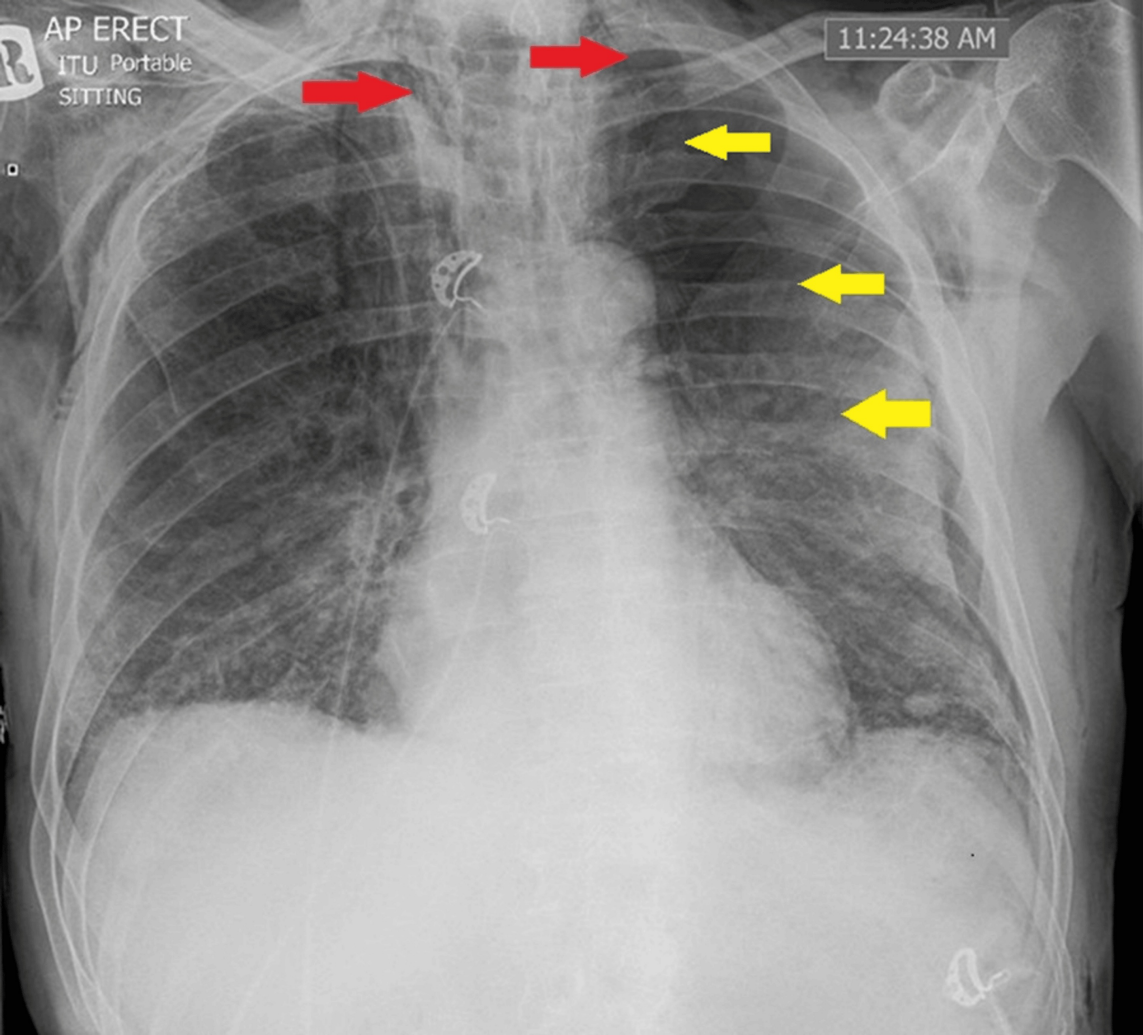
Portable chest X-ray showing subcutaneous emphysema (red arrows), pneumomediastinum, and left-sided pneumothorax (yellow arrows).
